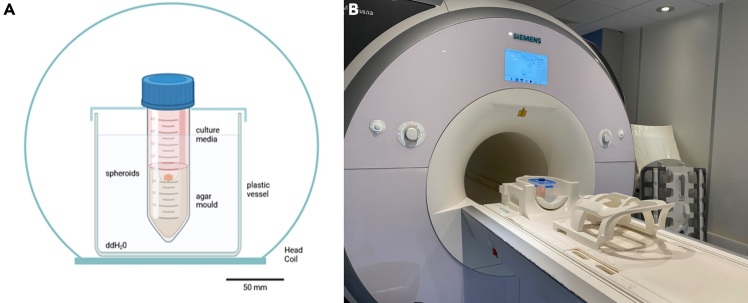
Experimental setup (A) Visualization of SW1353 cell spheroids in a mold of 1% agar and culture media, placed in a plastic box, surrounded by ddH_2_O and placed into the head coil of a 3 Tesla MRI scanner.^1^ (B) Placement within the scanner (MAGNETOM Prisma Fit).11.Move the table into the scanner.12.Start your imaging /MR protocols.13.Save your acquired data in DICOM-format.14.Remove the cultivation tube.15.Change the standard lid with the ventilated cap of the cell reactor tube.16.Store spheroids in an incubator (37°C, 5% CO_2_, normoxia) if further use or repeated examination is desired.*Note:* Double distilled H_2_O is used to ensure that there is absolutely no residue (e.g. rust) which might cause artefacts in imaging.*Note:* The box should stand upright and be fixed in such a way that no movement is possible during the measurement.CRITICAL: The temperature has a strong influence on MR features and should therefore be closely monitored and remain constant during subsequent measurements. Troubleshooting 2.
